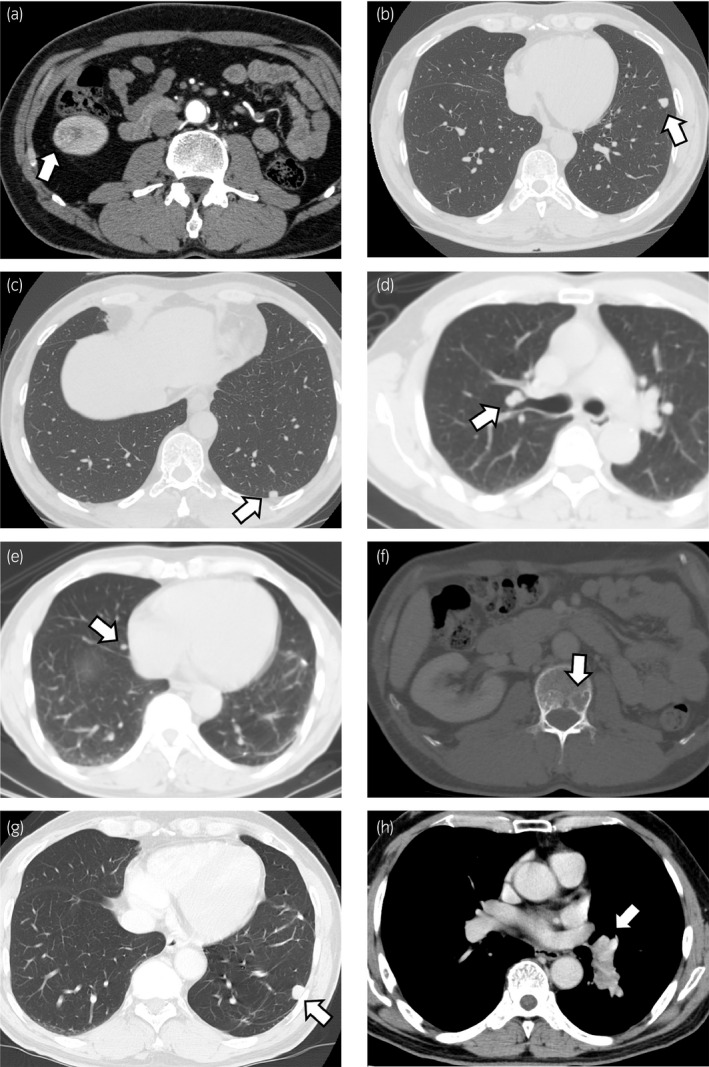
The CT images are shown. An arrow indicates a metastatic lesion. (a) 16‐mm enhanced mass in the lower pole of right kidney; (b) 9‐mm coin lesion in the upper lobe of left lung; (c) 8‐mm coin lesion in the lower lobe of left lung; (d) 8‐mm coin lesion near the hilum of right lung; (e) 5‐mm coin lesion in the middle lobe of right lung; (f) 15‐mm osteolytic lesion in the second lumbar spine; (g) 13‐mm coin lesion in the lower lobe of left lung; (h) 42‐mm left hilar lymph node.
